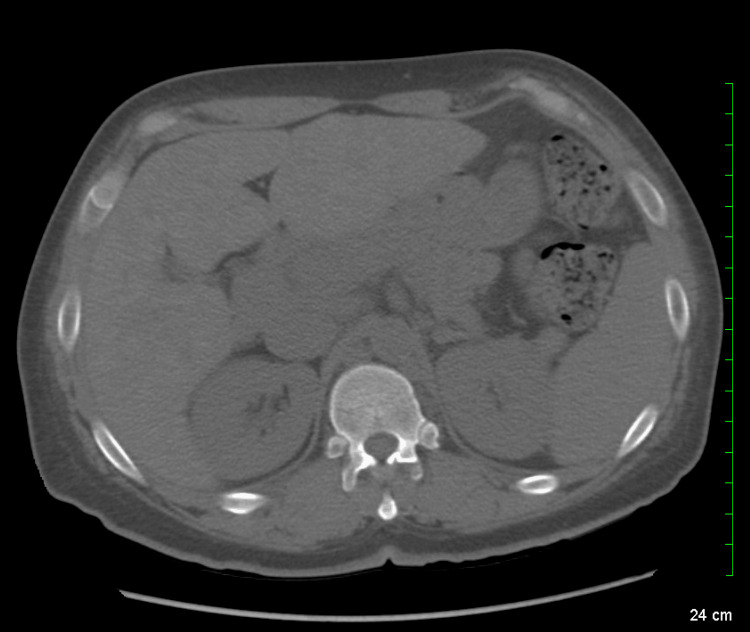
A CT scan of the abdomen without contrast; diffuse heterogenous attenuation of the liver is noted.
